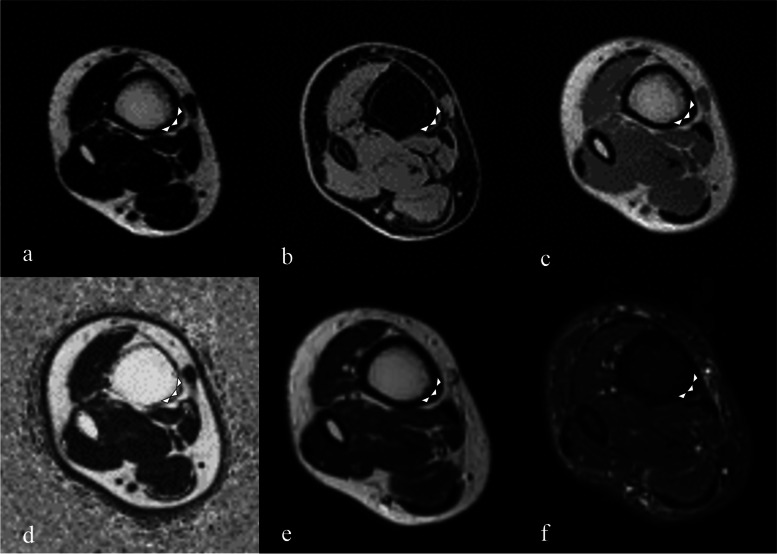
A 23-year-old man. Images in transverse plane were acquired 6 cm from the distal tip of the medial malleolus. a: Fat-only image obtained using LAVA-Flex. b: Water-only image obtained using LAVA-Flex. c: In-phase image (same T1-weighted imaging) obtained using LAVA-Flex. d: Fat fraction image obtained using Ideal IQ. e: T2-weighted image. f: short-tau inverse recovery (STIR). Adipose tissue positioned on the posteromedial tibial border (white arrowheads). The adipose tissue shows high signal intensity on fat-only, in-phase, fat fraction, and T2-weighted images. On the other hand, the adipose tissue shows low signal intensity on water-only and STIR images
